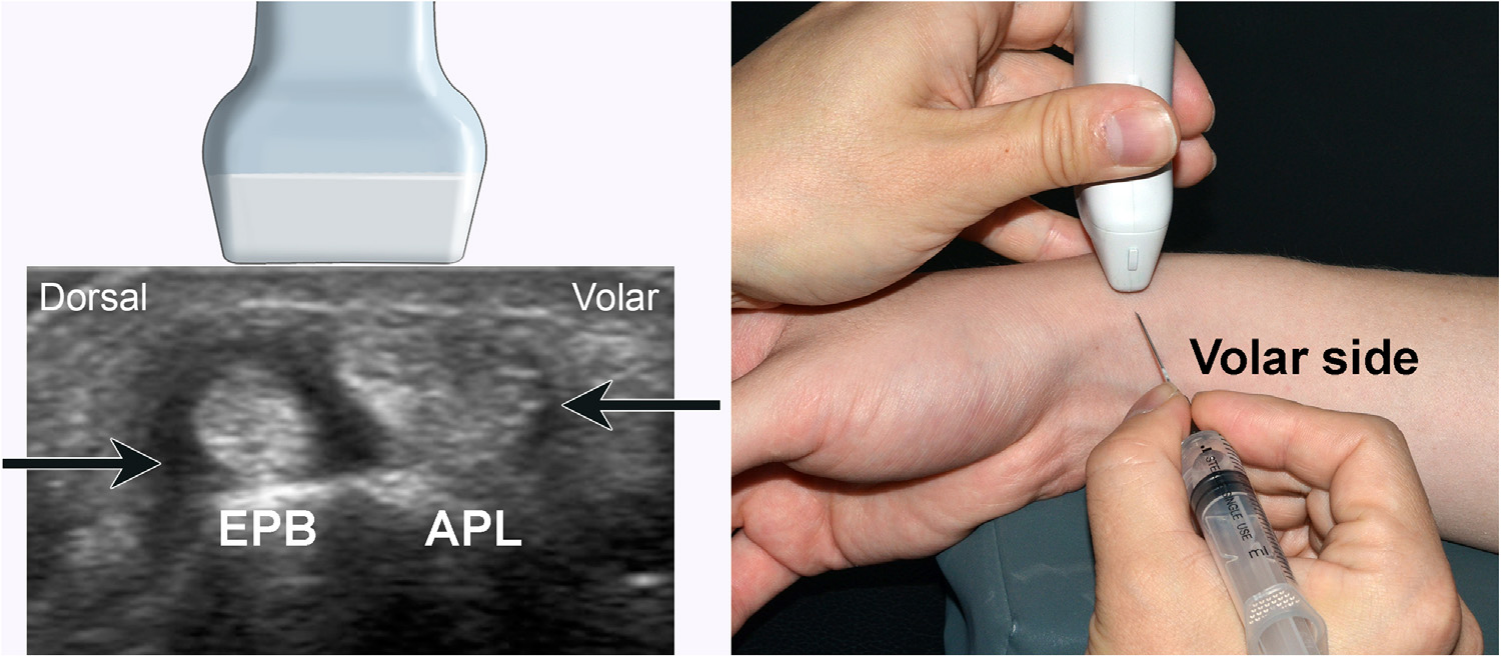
Ultrasound-guided steroid injection in De Quervain's disease. A. An ultrasound probe was located vertically along the path of the abductor pollicis longus muscle (APL) and extensor pollicis brevis muscle (EPB) to provide a short-axis view of the tendon. The needle for local steroid injection could be introduced from either the dorsal or volar side (arrow). B. This image shows the actual practice of observing the short-axis view using the probe, with the needle approaching from the volar side.
